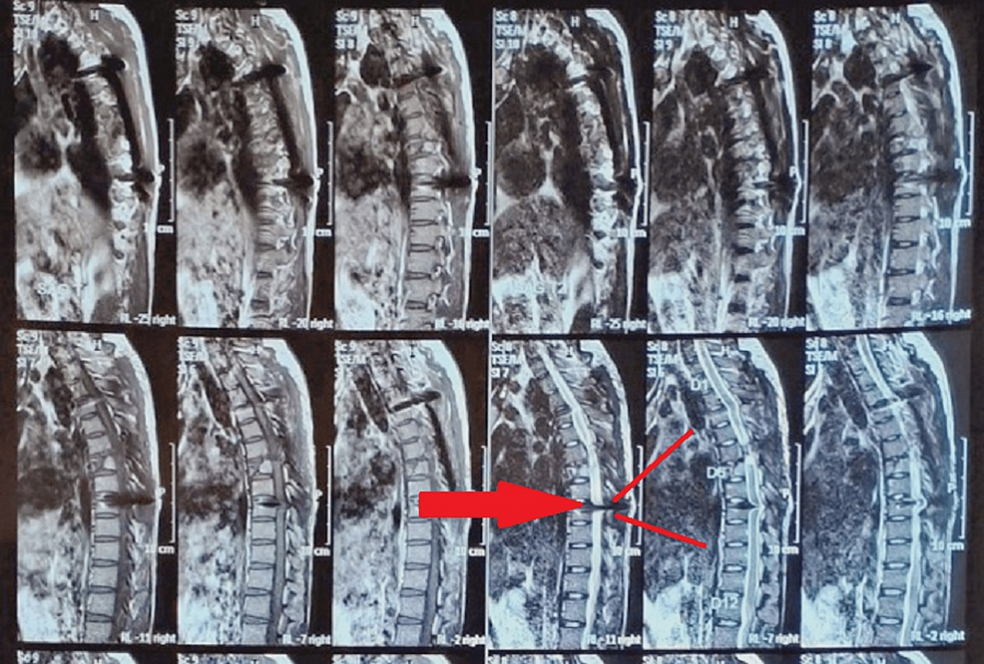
Magnetic resonance image of the patient The red arrow and lines indicate the level of injury.
